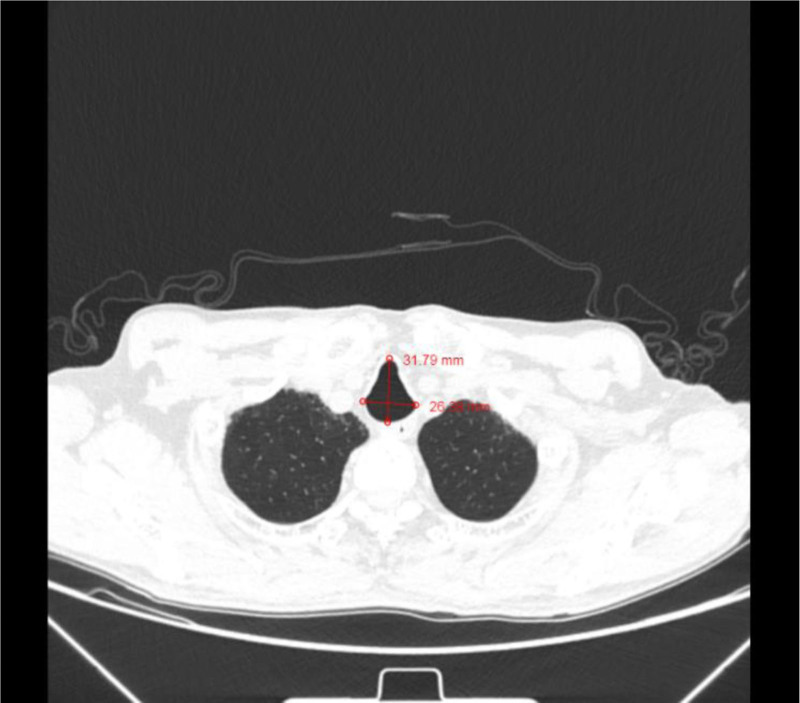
Chest CT imaging demonstrated sagittal and coronal diameters measuring 31.79 and 26.39 mm, respectively, at the maximum transverse cross-section of the tracheal lumen. CT = computed tomography.
